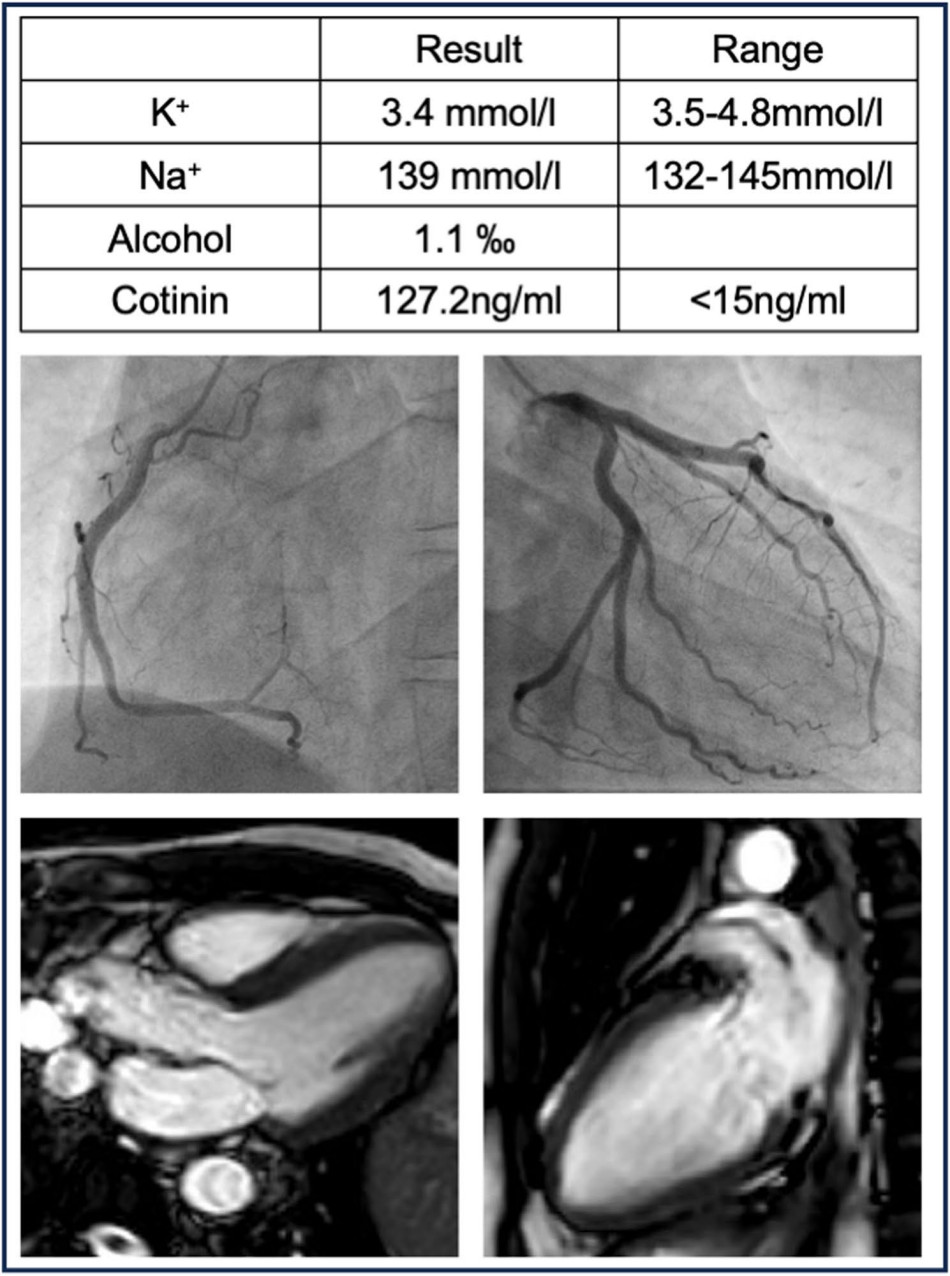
Patient´s laboratory findings (upper row), coronary angiography (middle) and cardiac MRI (bottom)
